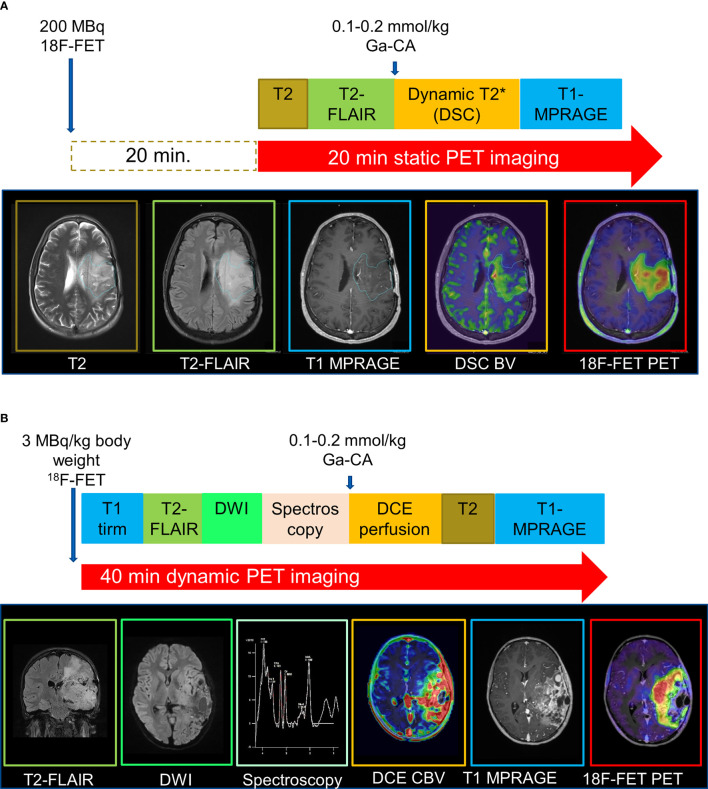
Examples of hybrid PET/MRI protocols. MRI data were acquired during acquisition of (A) static 20-minute or (B) dynamic 40-minute PET data. Adapted with permission from (103) and (104), respectively.
